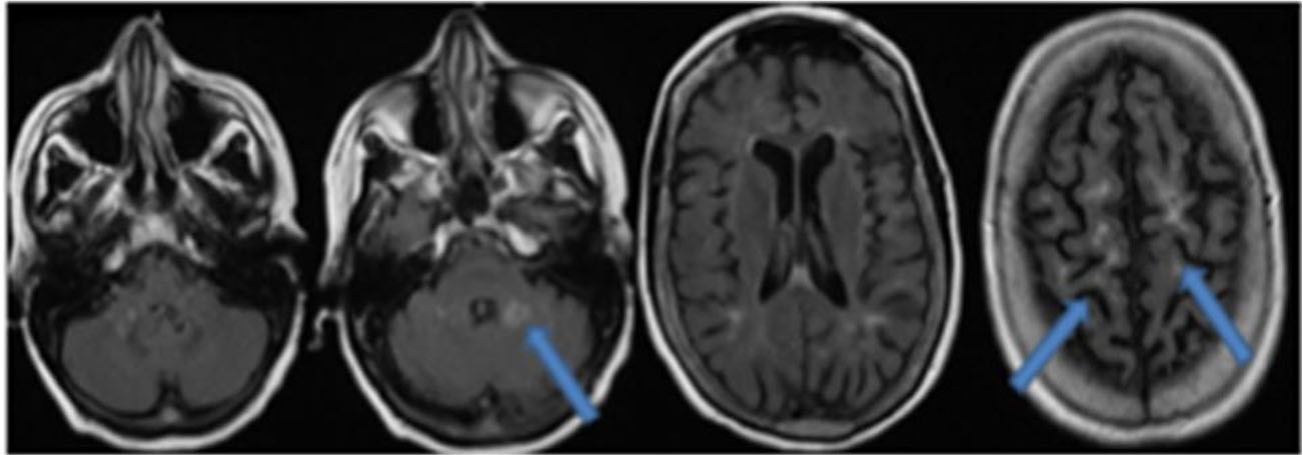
Case two, 2012: FLAIR MRI sequences showing new lesions in the posterior fossa (see *arrow*) and an increase in the number of lesions in the high frontoparietal white matter bilaterally (see *arrows*). There was also increased cerebral atrophy. There was no mass effect, perilesional oedema, or enhancement after contrast administration (not shown)
